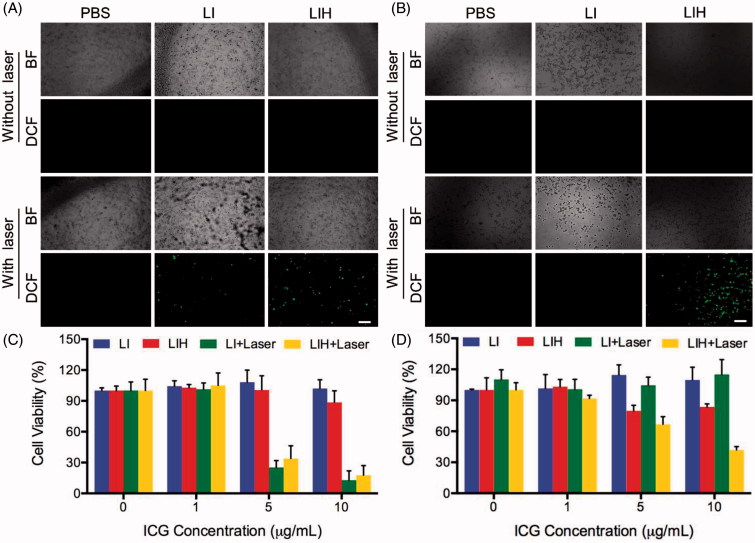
PDT efficiency of LIH. Intracellular ROS detection of PBS, LI, and LIH under (A) normaxia and (B) hypoxia condition with or without laser irradiation (808 nm, 1 W/cm^2^, and 1 min). Cytotoxicity of LI and LIH against CT-26 cells in (C) normaxia and (D) hypoxia environment without or with laser irradiation (808 nm, 1 W/cm^2^, and 1 min).
